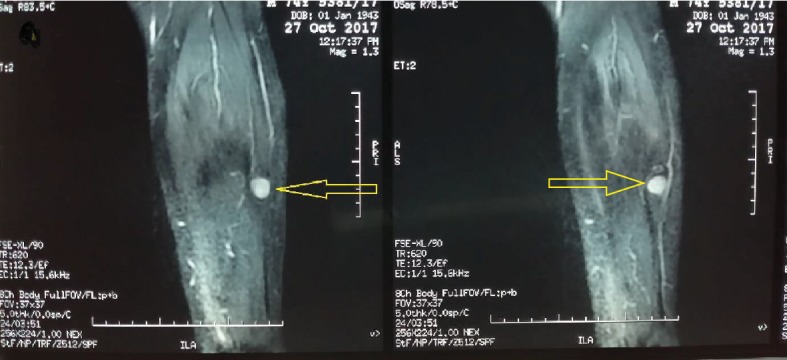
MRI T1 fat suppression showed the tumor mass (arrows).
